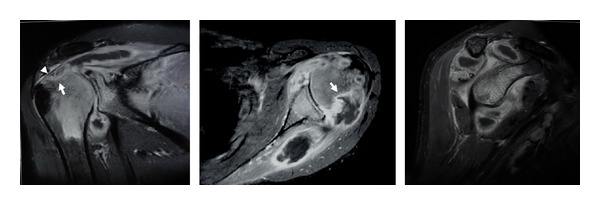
MRI demonstrating a large abscess surrounding the humeral head and extending into the supraspinatus and infraspinatus muscle substance. Significant bone erosion in the superior portion of the humeral head (white arrow) and thinning of the supraspinatus tendon (white arrow head) were revealed.
